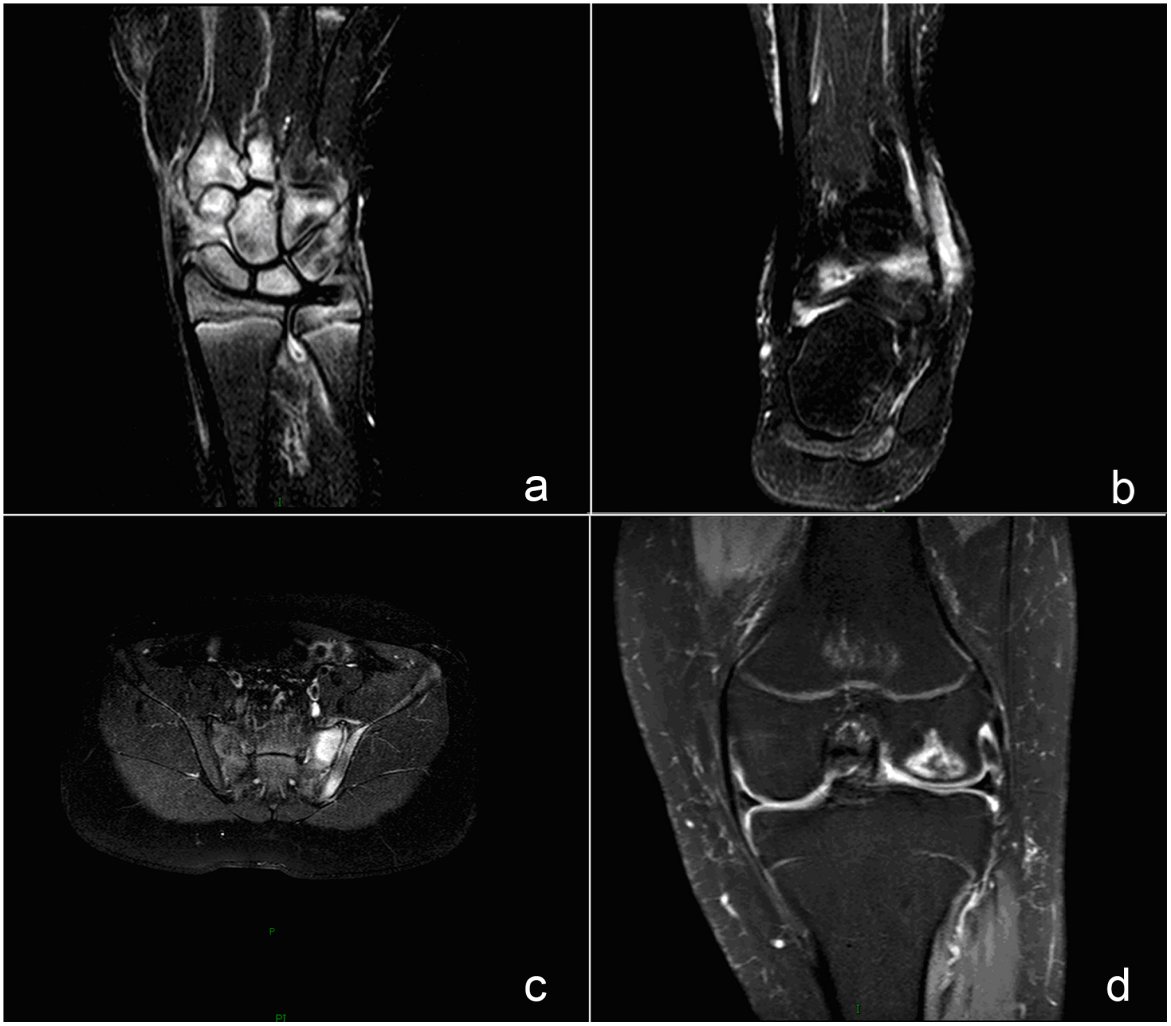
MRI findings of frequently involved joints. (a, b, c and d) Bone marrow edema (BME) appears as hyperintense signal in SPAIR sequence, reflecting active inflammatory lesion. a SPAIR image of wrist demonstrates BME on the carpal. b SPAIR image of a ankle shows BME at the tibiofibula. c SPAIR image of sacroiliac joint shows BME on the left of sacroiliac joint. d SPAIR image of a knee joint shows BME at the distal femur.
